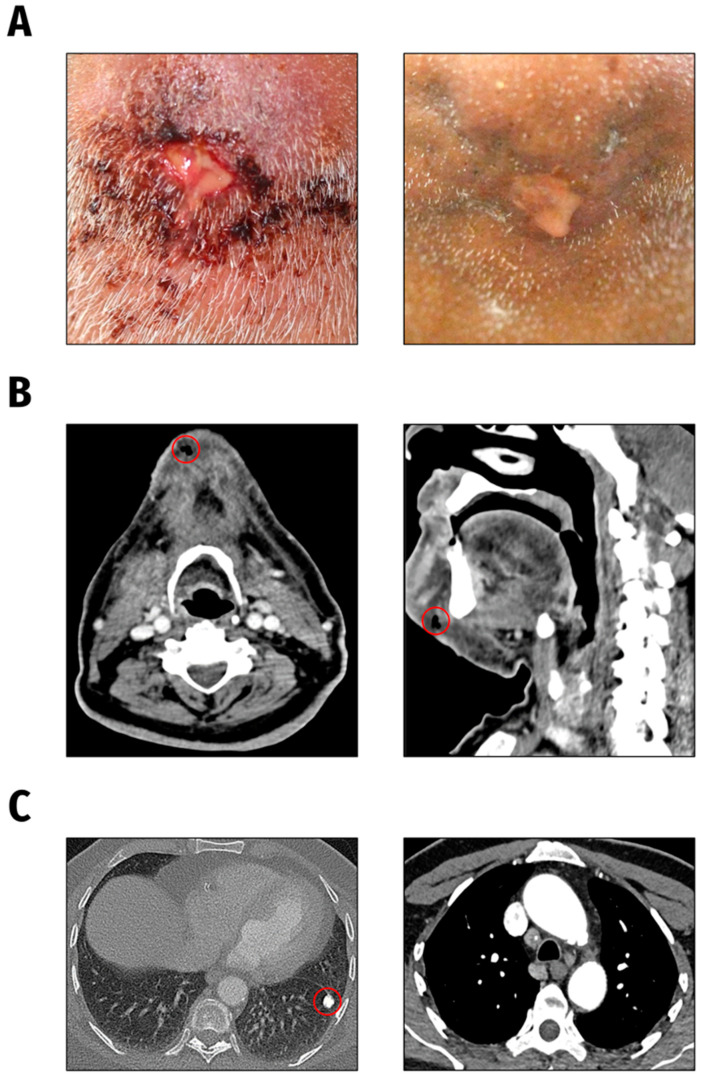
Clinical presentation. (A). Submental wound findings at initial presentation (left) and following antibiotic treatment one month later (right). (B). CT head and neck findings: subcutaneous air accumulation and circumscribed fluid in the chin region. (C). CT chest scan findings: calcified focal lesion in the left lung as well as mediastinal and pulmonary lymphadenopathy.
